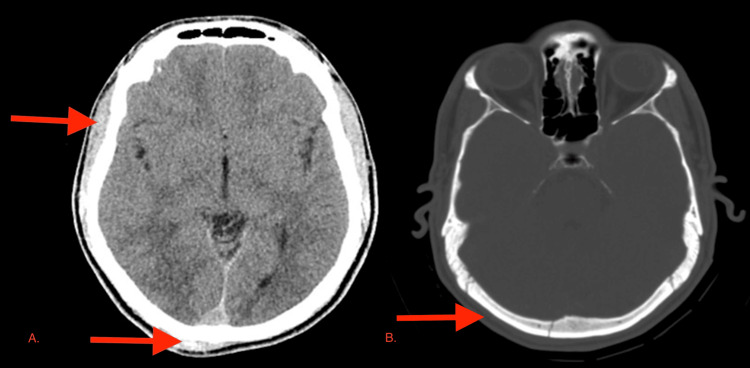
Initial CT brain showed (A) small hemorrhagic contusions in the right inferior-posterior temporal and right occipital areas and (B) non-depressed fracture of the right occipital bone CT: computed tomography
